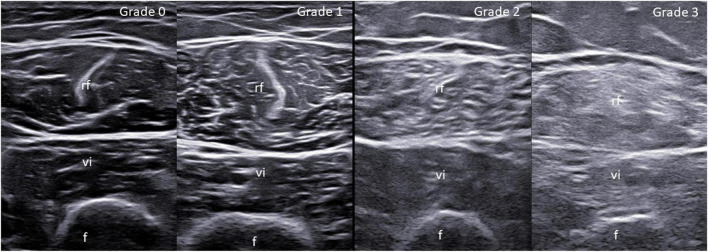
Visual semi-quantitative scale for the assessment of muscle echogenicity. Ultrasound (US) transverse scan images of the quadriceps muscle obtained at the midpoint between the anterior superior iliac spine and the upper pole of the patella. Grade 0 = normal (i.e., normal hypoechoic muscle); Grade 1 = mild (homogeneously distributed overall increase of the echogenicity involving ≤ one-third of the entire muscle tissue); Grade 2 = moderate (homogeneously distributed overall increase of the echogenicity involving > one-third but ≤ two-thirds of the entire muscle tissue); Grade 3 = severe (homogeneously distributed overall increase of the echogenicity involving > two-thirds of the entire muscle tissue). f, femur; rf, rectus femoris muscle; vi, vastus intermedius muscle.
